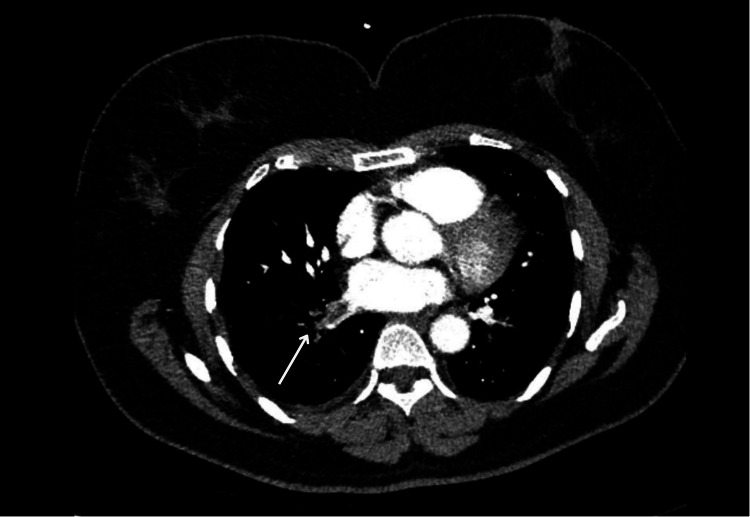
Axial CT pulmonary angiography (CTPA) images from Case 2 demonstrating a large filling defect within the right inferior pulmonary vein extending from segmental and subsegmental branches of the right lower lung lobe (arrow)
